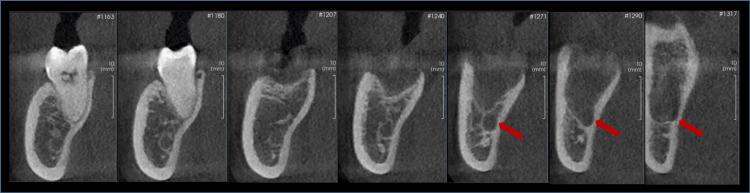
Cone beam tomography; sagittal section showing a hypodense image corresponding to the alveolus, with proximity to and communication with the inferior alveolar nerve (red arrows).
